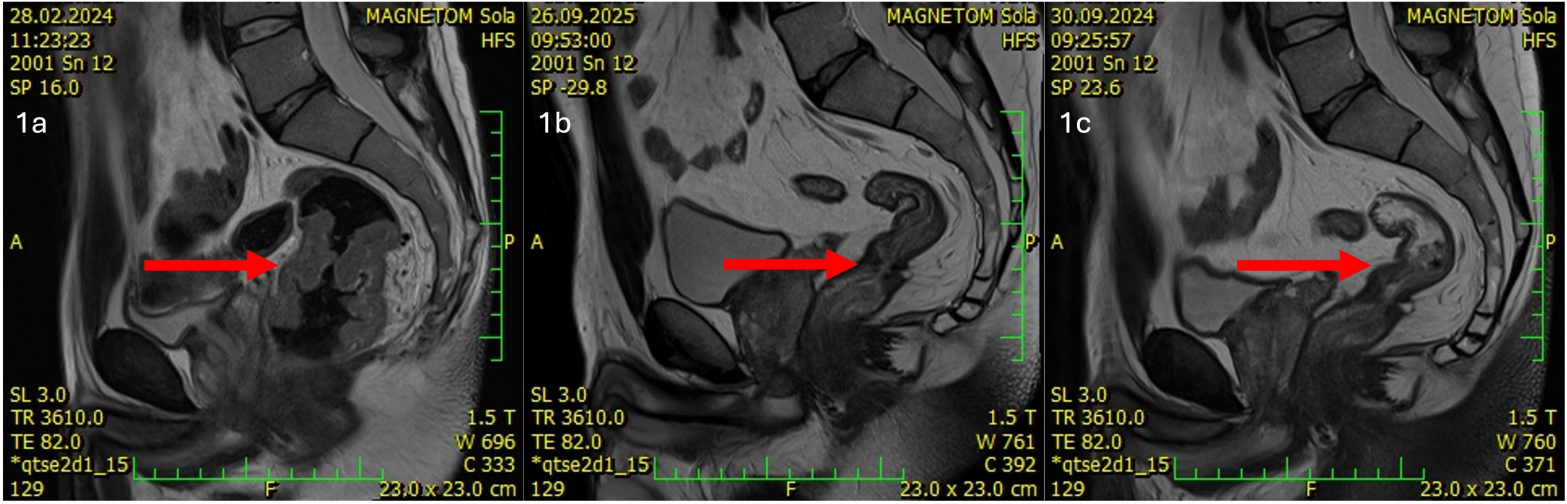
(a) Disease status prior to initiation of immunotherapy (magnetic resonance imaging, red arrow showing primary tumor) (b) Disease status at the end of immunotherapy (cCR) (magnetic resonance imaging, red arrow showing cCR of primary tumor). (c) Disease status 12 months after the end of immunotherapy (persistent cCR) (magnetic resonance imaging, red arrow showing cCR of primary tumor).
